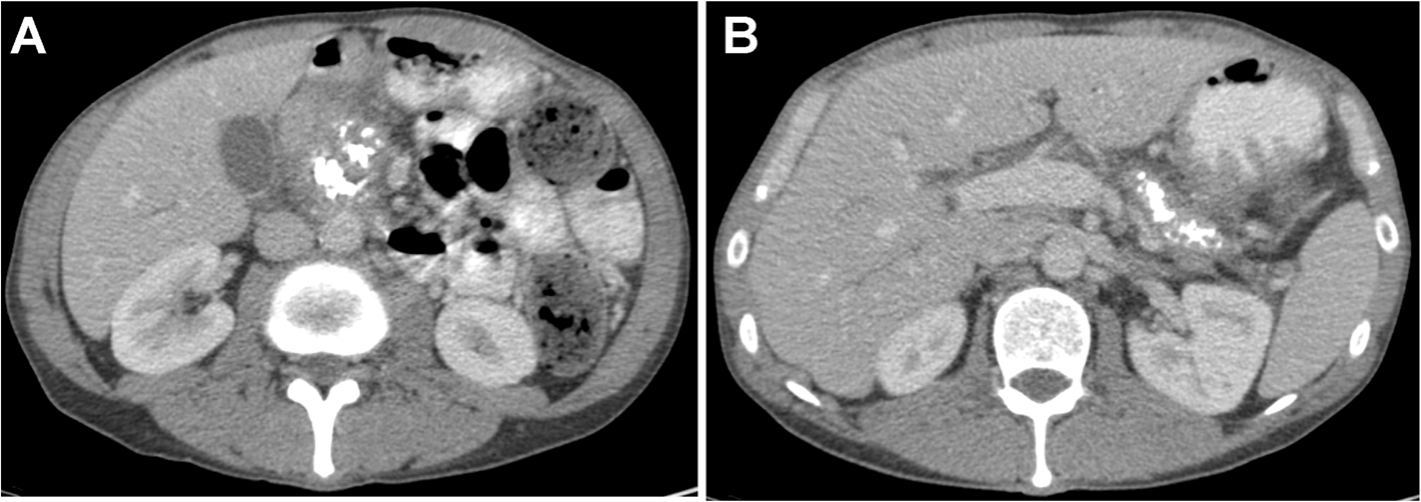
CT scan depicting fully impacted stones casting the entire main pancreatic duct from head (A) to tail (B).
